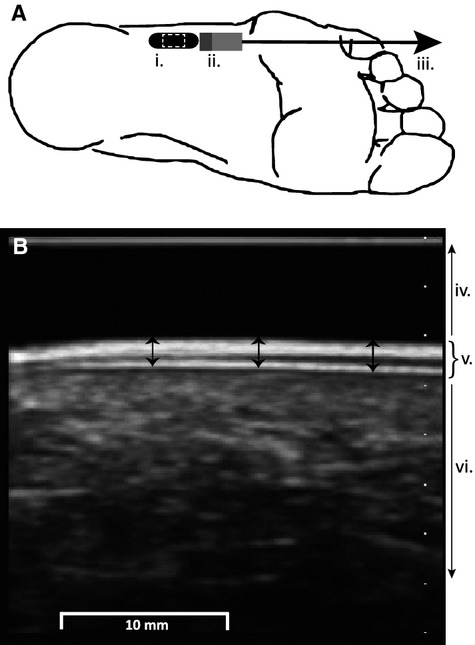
(A) Ultrasound and skin stretch set up. Ultrasound transducer location (i.) for the LatArch test site, with the image site outlined by a white dashed line. A plastic tab (ii.) glued to the skin facilitated 10 mm of pull (iii.). (B) B-mode ultrasound image including the standoff pad (iv.), epidermis (v.) and dermis plus subcutaneous tissue (vi.). Three measurements of epidermal thickness (arrows) were taken and averaged at each test site.
